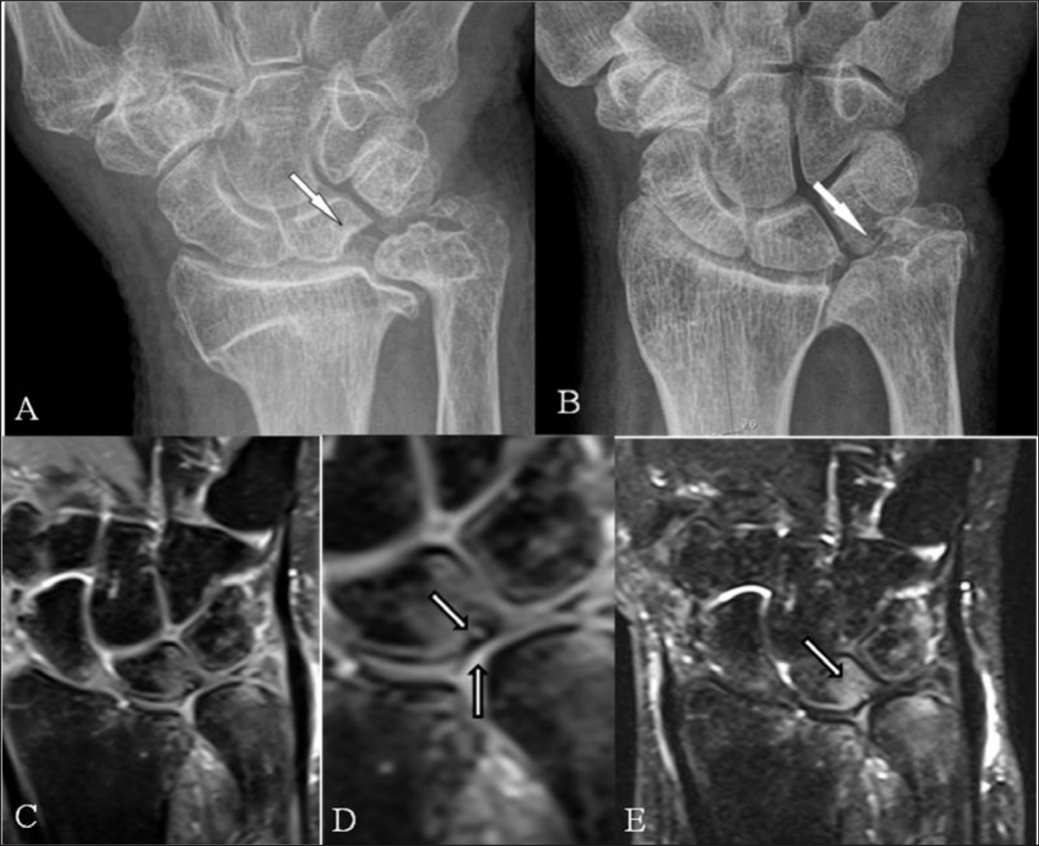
Ulnolunate and/or ulnotriquetral abutment. (A, B) PA plain radiographs; (C, D) Coronal SE PD-WI FS; and (E) Coronal SE T2-WI FS. (A) Ulnolunate abutment with a sclerotic defined impression at the ulnar side of the lunate bone. (B) Ulnotriquetral abutment with sclerotic bordered neoarticulation. (C–E) Ulnolunate abutment with chondromalacia at the ulnar border of the lunate bone (D, vertical arrow), subchondral cyst (D, oblique arrow), and bone marrow oedema centered at the ulnar side of the lunate bone (E).
